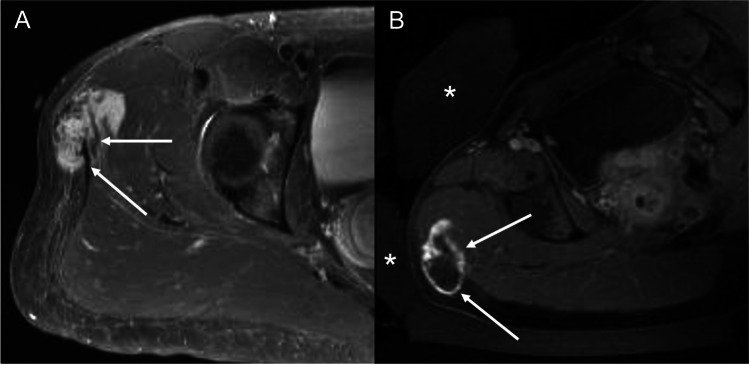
Desmoid tumor. A Pre-treatment axial T1-weighted fat-saturated post-contrast sequence obtained prior to MRgFUS demonstrates a homogeneously enhancing mass in the lateral hip superior to the greater trochanter, compatible with a biopsy-proven desmoid tumor (white arrow). B Post-treatment axial T1-weighted fat-saturated post-contrast sequence performed immediately following MRgFUS exhibits 85% ablation area with minimal residual rim enhancement (white arrows). Water bags (asterisks) are positioned anterior and lateral to the right hip to minimize the risk of skin injury
